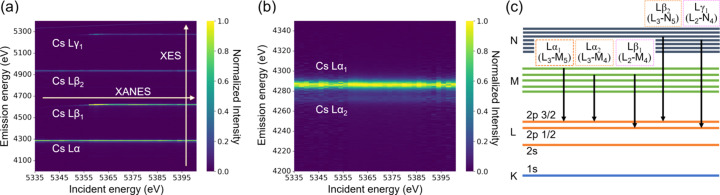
(*a*) 2D image of the intensity of emitted X-rays, normalized by the maximum intensity of La_1_, as a function of incident and emission X-ray energies for CsNO_3_. (*b*) Enlarged 2D map of part (*a*) to visualize the *L*α_1_ and *L*α_2_ features. (*c*) The transitions of the detected emission lines and their Siegbahn notations (*e.g.L*α_1_) and IUPAC notations (*e.g.L*_3_–*M*_5_).
